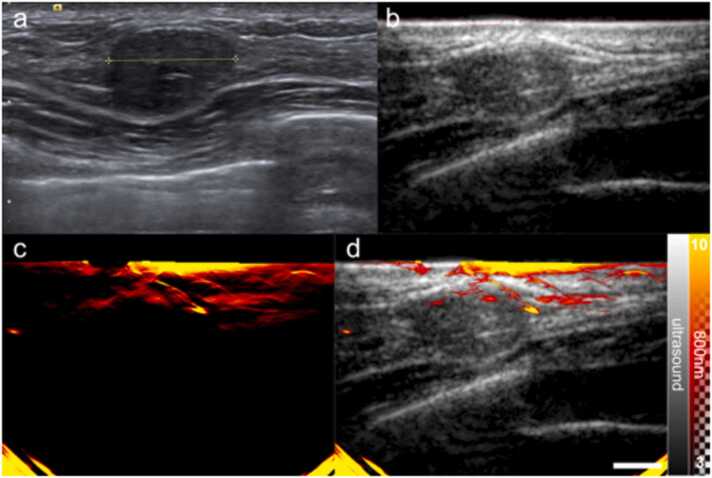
Exemplar fibroadenoma (benign). (a) Standard-of-care ultrasound reveals an oval hypoechoic mass, without lobulations; the mass is greater in width than height. (b) The US image from the OA-US scan also reveals an oval hypoechoic mass (c) The OA image from the OA-US scan at 800 nm reveals blood vessels that appear to be draped over the lesion. (d) Overlaid OA-US image highlights the relationship between the visualised blood vessels and the benign mass. Scale bar 1 cm.
